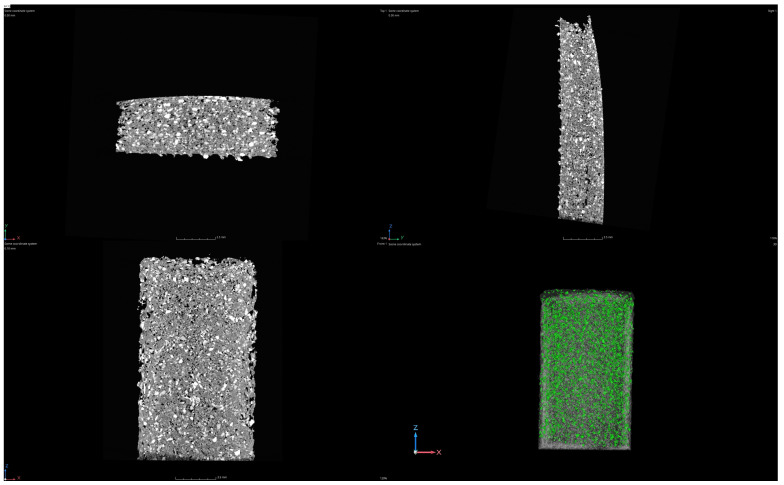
Images from CT for the 3D-printed samples obtained from PP70.
